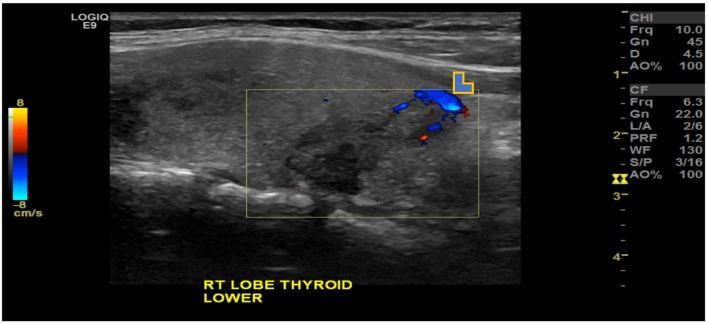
Thyroid ultrasound showing well-defined, mildly lobulated solid lesion posterior to the lower right thyroid lobe displaying peripheral vascularity (arrowhead).
